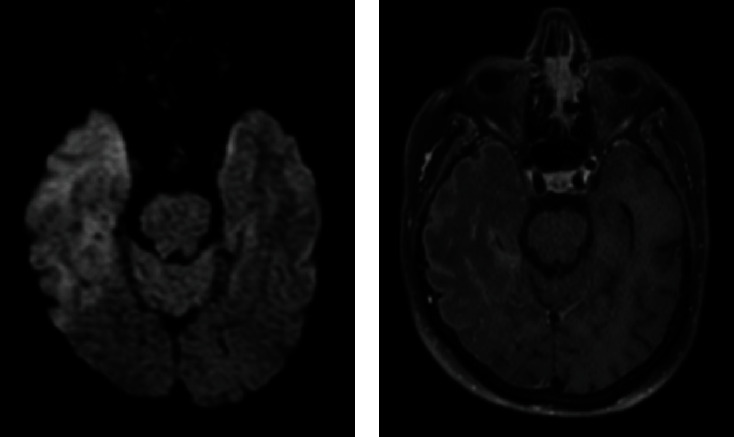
Right temporal lobe predominant encephalitis in a 58-year-old male patient (a, b). (a) Moderate diffusion restriction was observed in the DWI image. (b) In the postcontrast T1-weighted image, moderate enhancement in the subcortical and marked enhancement in the subpial subarachnoid were observed.
